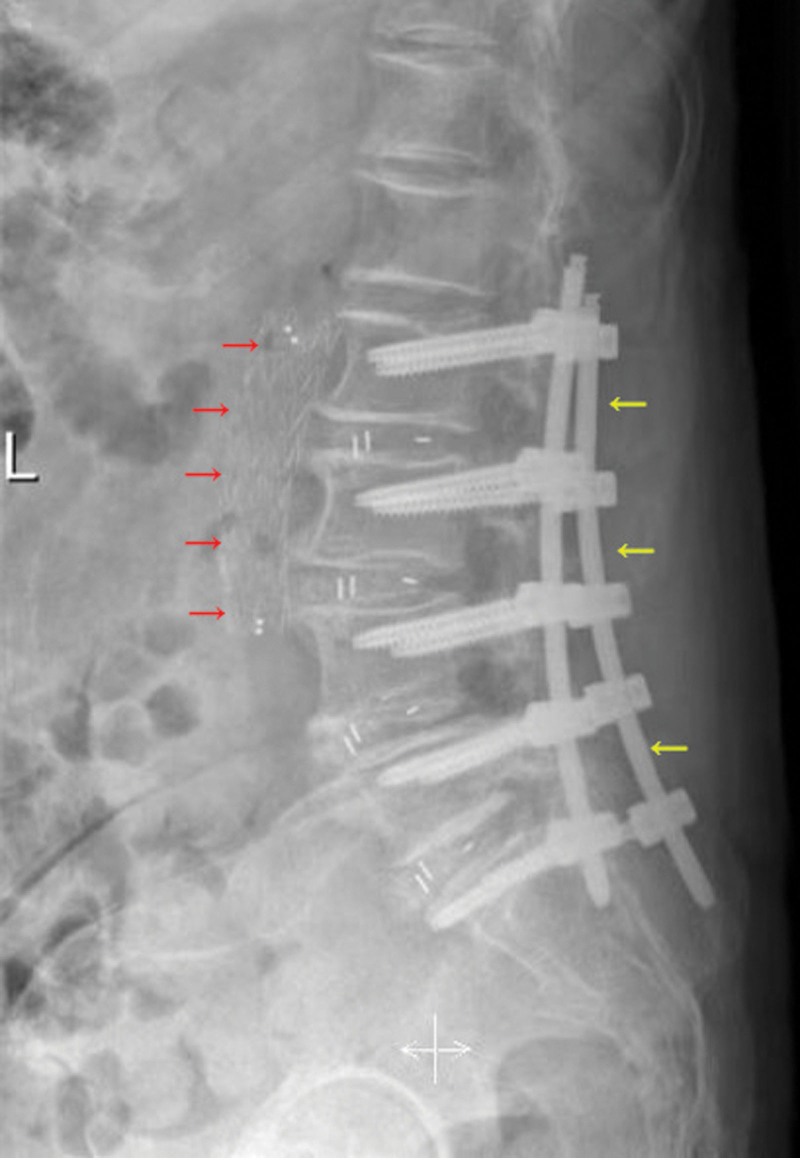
A postoperative lateral radiograph of the torso (red arrows) demonstrates a properly aligned stent inside the abdominal aorta. Yellow arrows indicate strong pedicle crew fixation from L1-S1.
